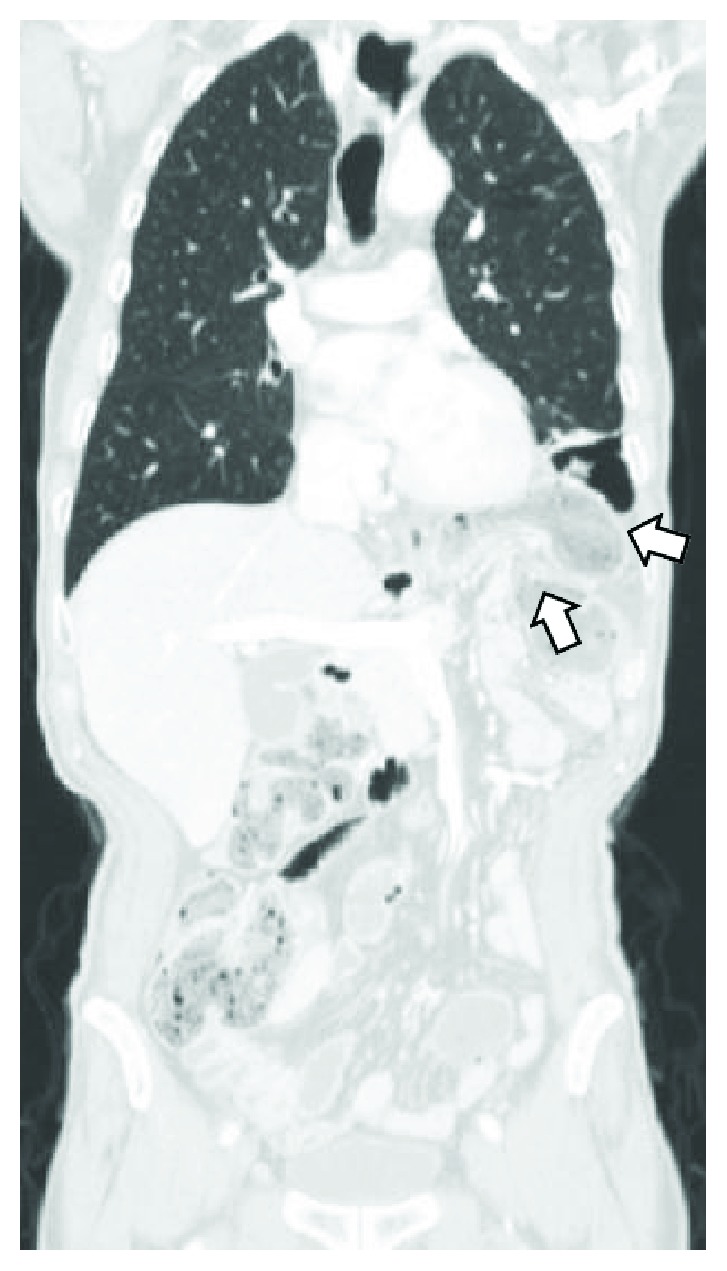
CT scan performed during the oncological follow-up. The diaphragmatic hernia was clearly visible but has not been described in the radiological report. Left side diaphragmatic defect (lower arrow); abdominal viscera in the left hemithorax (upper arrow).
